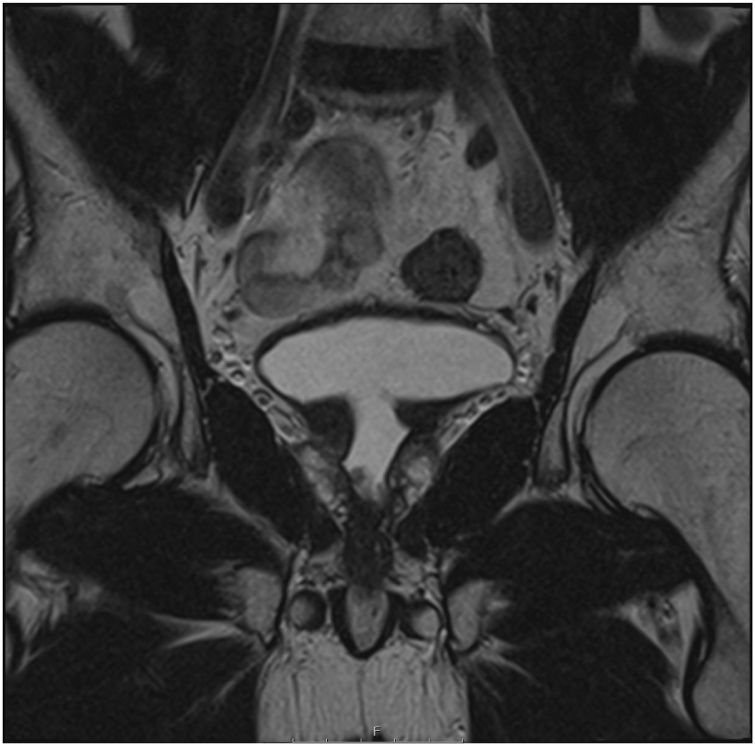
T2-weighted MRI scan (coronal view) shows no recurrence of the prostate after 1 year of the HoLEP.
